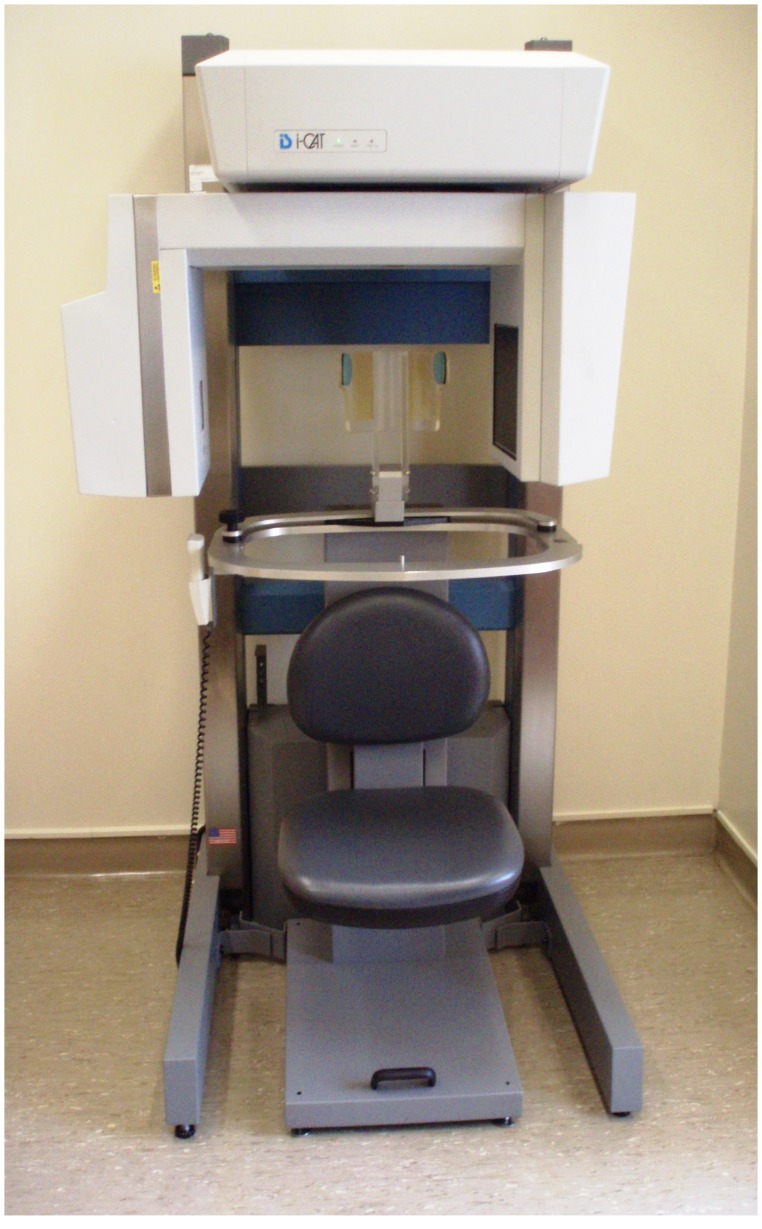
The i-CAT Cone-beam CT scanner.
